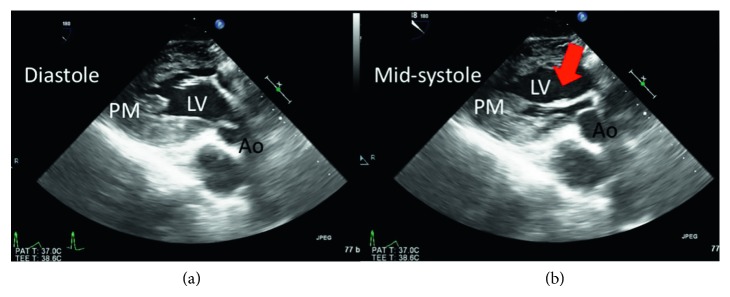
Long axis of the LV from a deep transgastric view using transesophageal echocardiography. LV = left ventricle, PM = posterior papillary muscle, and Ao = aorta. During midsystole, there is anterior motion of the apically displaced posterior papillary muscle (red arrow).
